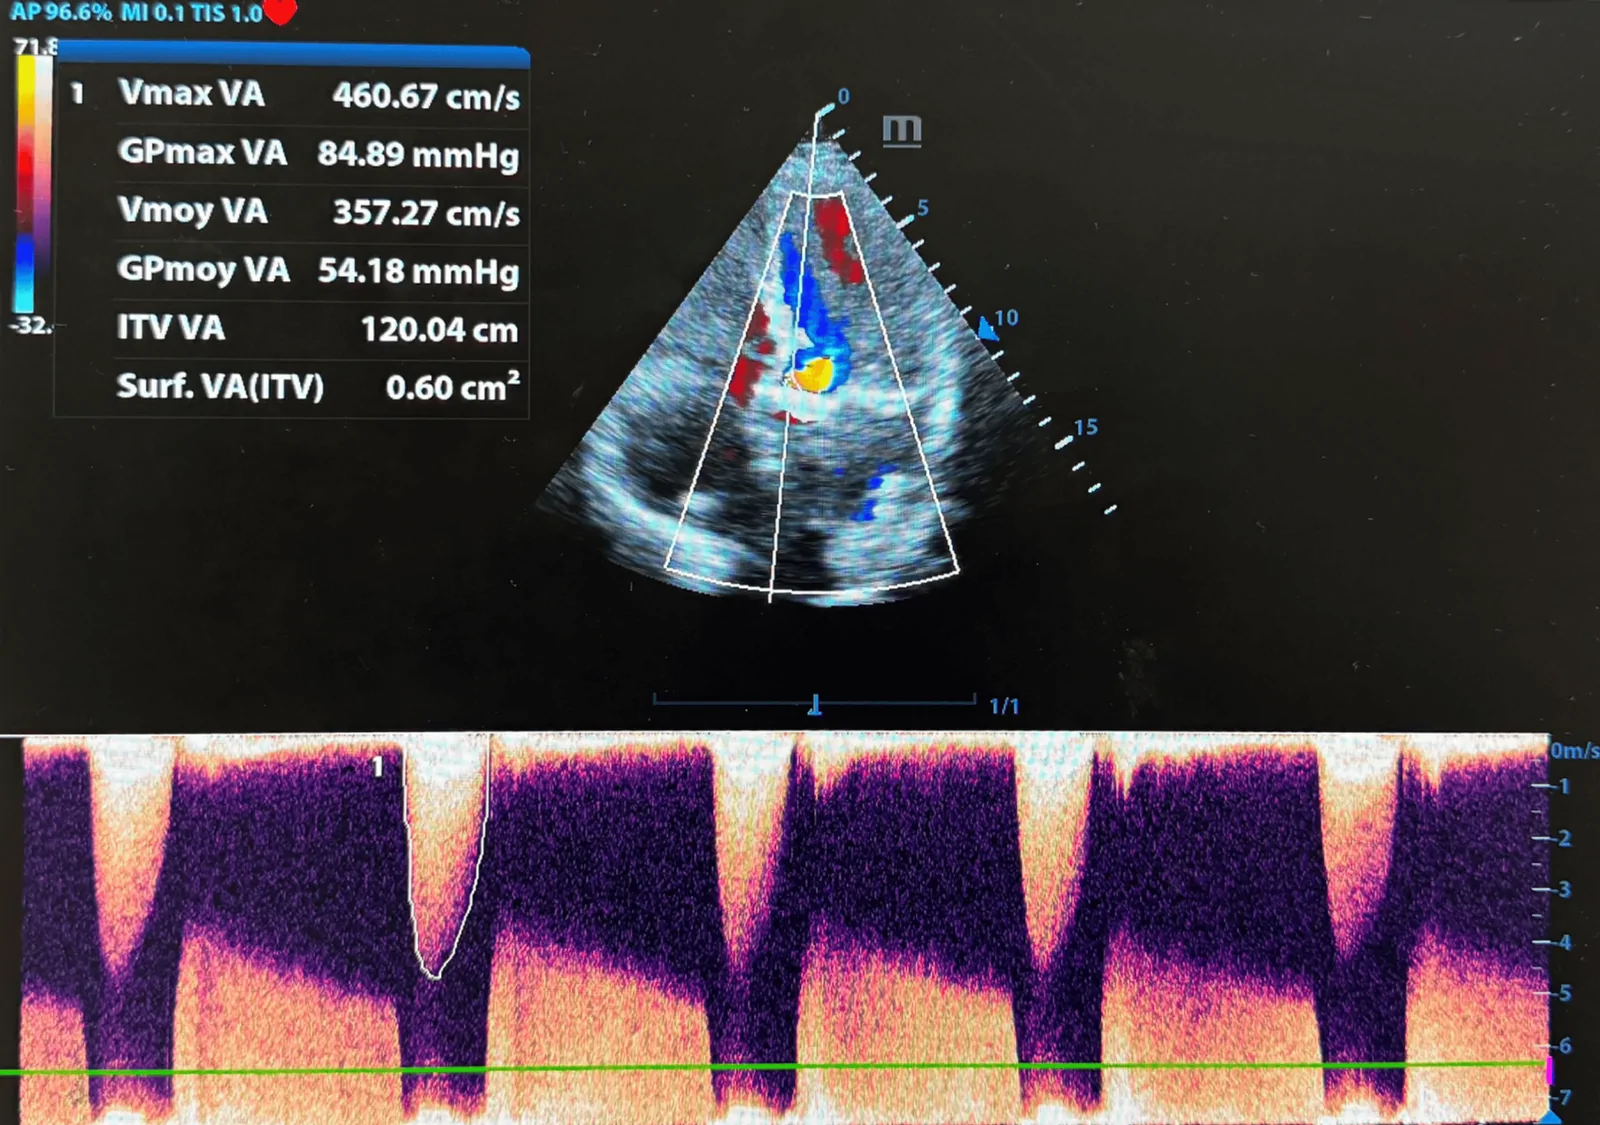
Echocardiographic assessment of aortic stenosis in Case 2. Continuous-wave Doppler echocardiography demonstrating severe aortic stenosis, with a peak aortic jet velocity of 4.61 m/s, a mean transvalvular gradient of 54.18 mmHg, and a calculated aortic valve area of 0.60 cm².
